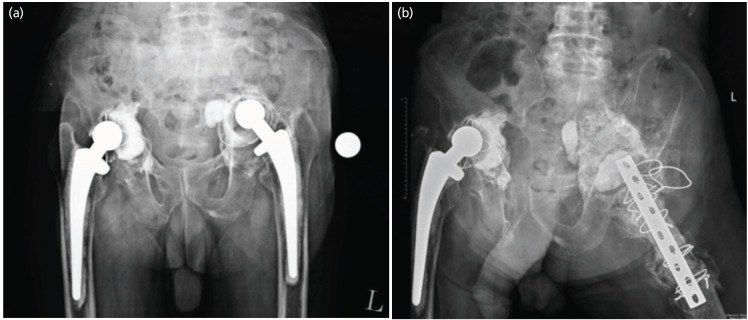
(a) AP Pelvic radiograph showing bilateral acetabular cup loosening with medial and superior protrusion of the acetabulum. (b) AP Pelvic radiograph showing AIBG integration.
